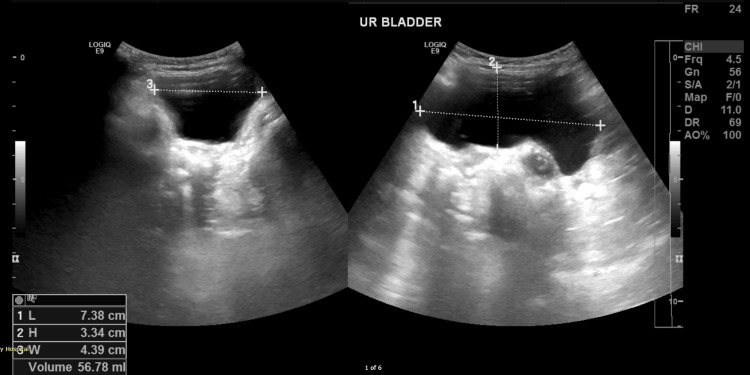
Ultrasound of the bladder. The urinary bladder before discharge is well distended (56 mL), showing normal wall thickness and no obvious intraluminal abnormality.
